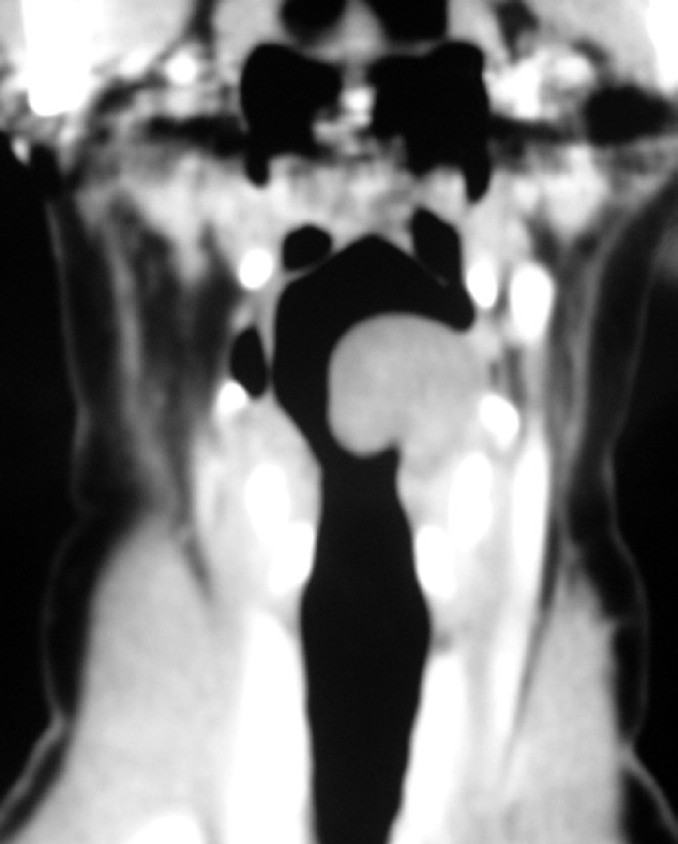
Computed tomography scan showing a well-defined homogeneous mass in the left arythenoid
